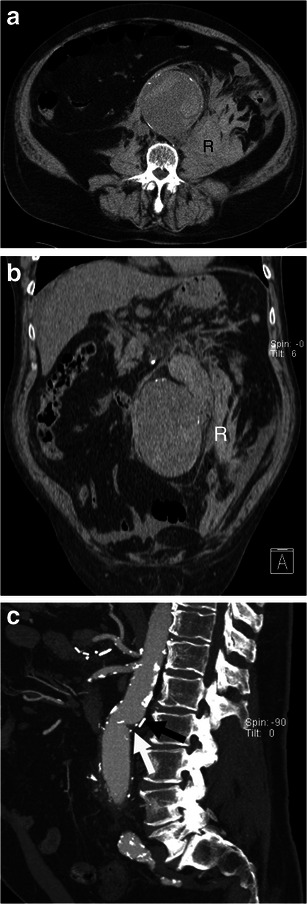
Open surgical repair. a Axial and b coronal unenhanced CT image of a 73-year-old man demonstrates ruptured AAA with a retroperitoneal haematoma (*R*). c Sagittal enhanced CT image of the same patient after successful open surgical repair shows the junction between the native aorta and aortic graft (*white arrow)* and a surgical clip (*black arrow*)
